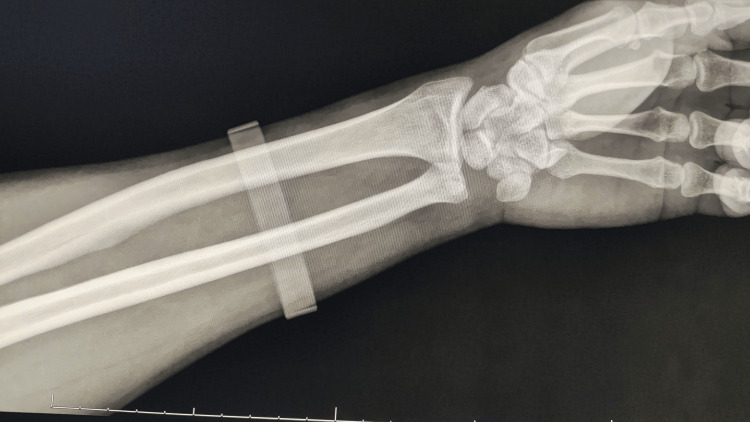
Anteroposterior wrist X-ray Anteroposterior wrist radiograph showing suboptimal positioning and poor visualization of the ulnar styloid.
